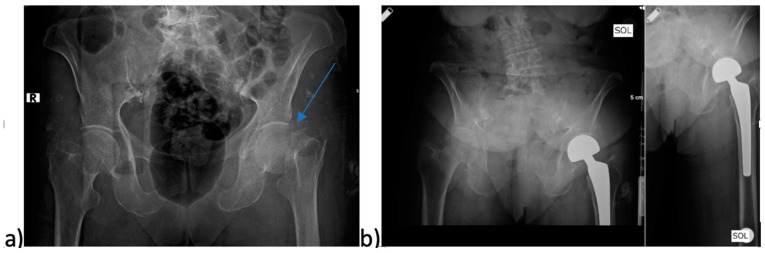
An 83-year-old female patient diagnosed with a Garden Type 4 fracture in the area indicated by the arrow in the left femoral neck, who underwent bipolar hemiarthroplasty and was mobilized on the second postoperative day. (a) Preoperative pelvis anteroposterior X-ray images; the fracture line is marked with the arrow. (b) Postoperative pelvis and left hip anteroposterior X-ray images.
